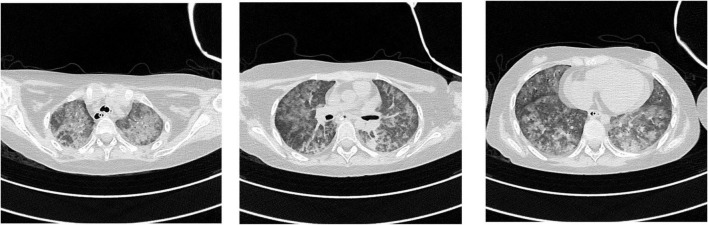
Chest computed tomography (CT) of the patient (10 days after admission). Extensive ground-glass opacities were observed in both lungs.
